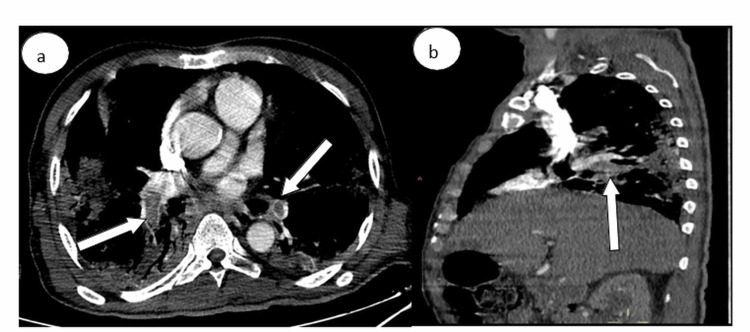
Computed tomography pulmonary angiography in (a) axial and (b) sagittal windows showing a bilateral pulmonary embolism (white arrows) of the two pulmonary arteries extended to the lobar branches
